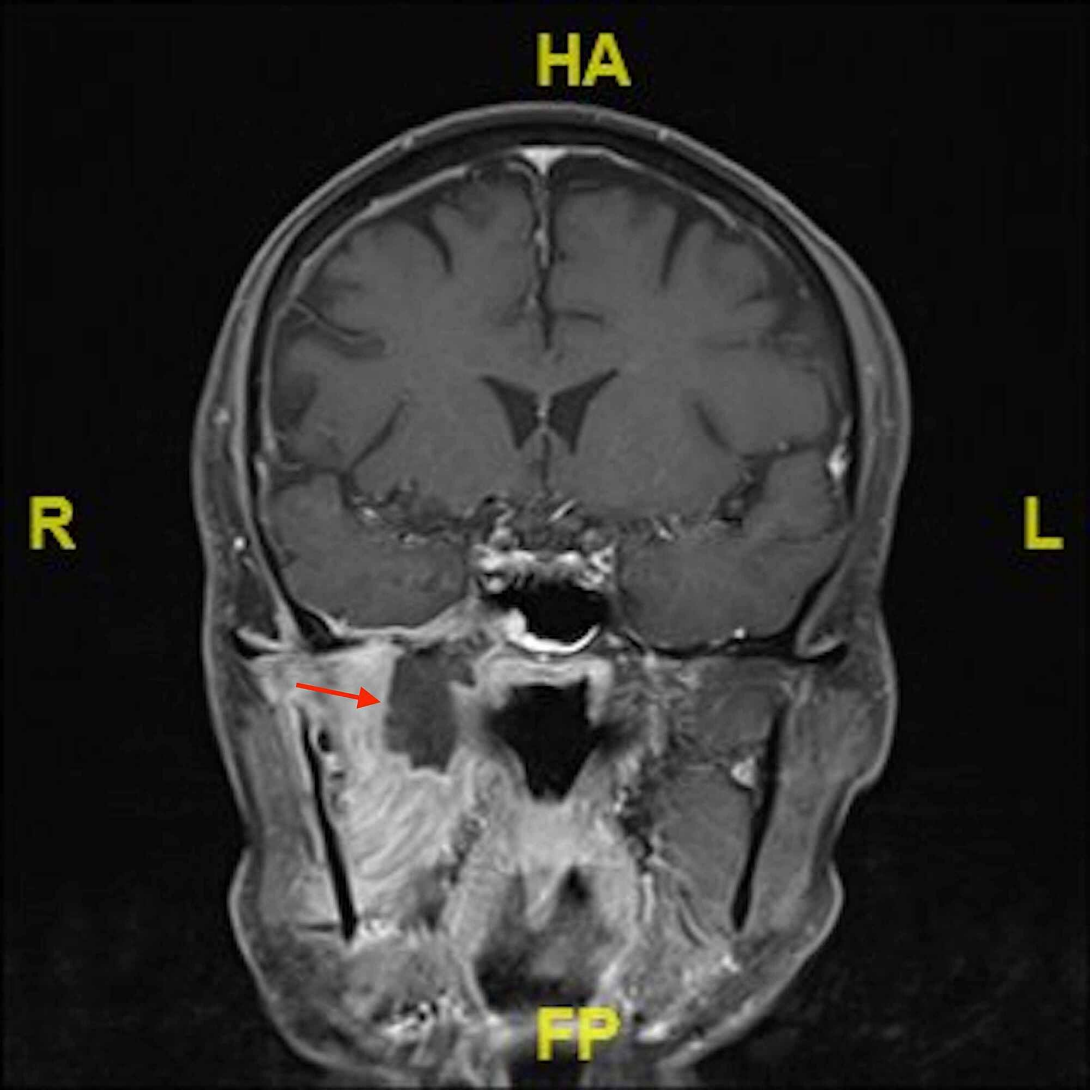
MRI scan demonstrating the hypointense lesion at the time of presentation as seen in T2 (transverse relaxation time) MRI: magnetic resonance imaging
